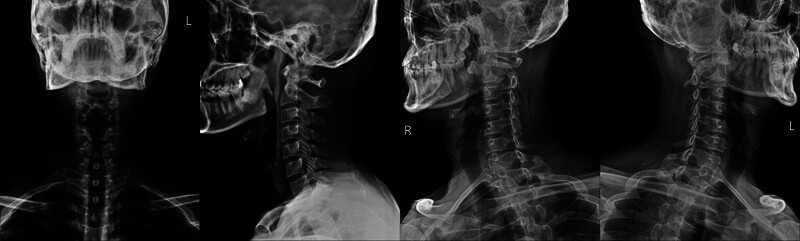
X-ray image scan of the cervical spine. C-spine series (anterior to posterior, lateral, oblique) was taken, and no remarkable findings were found other than straightening of cervical spine curvature.
